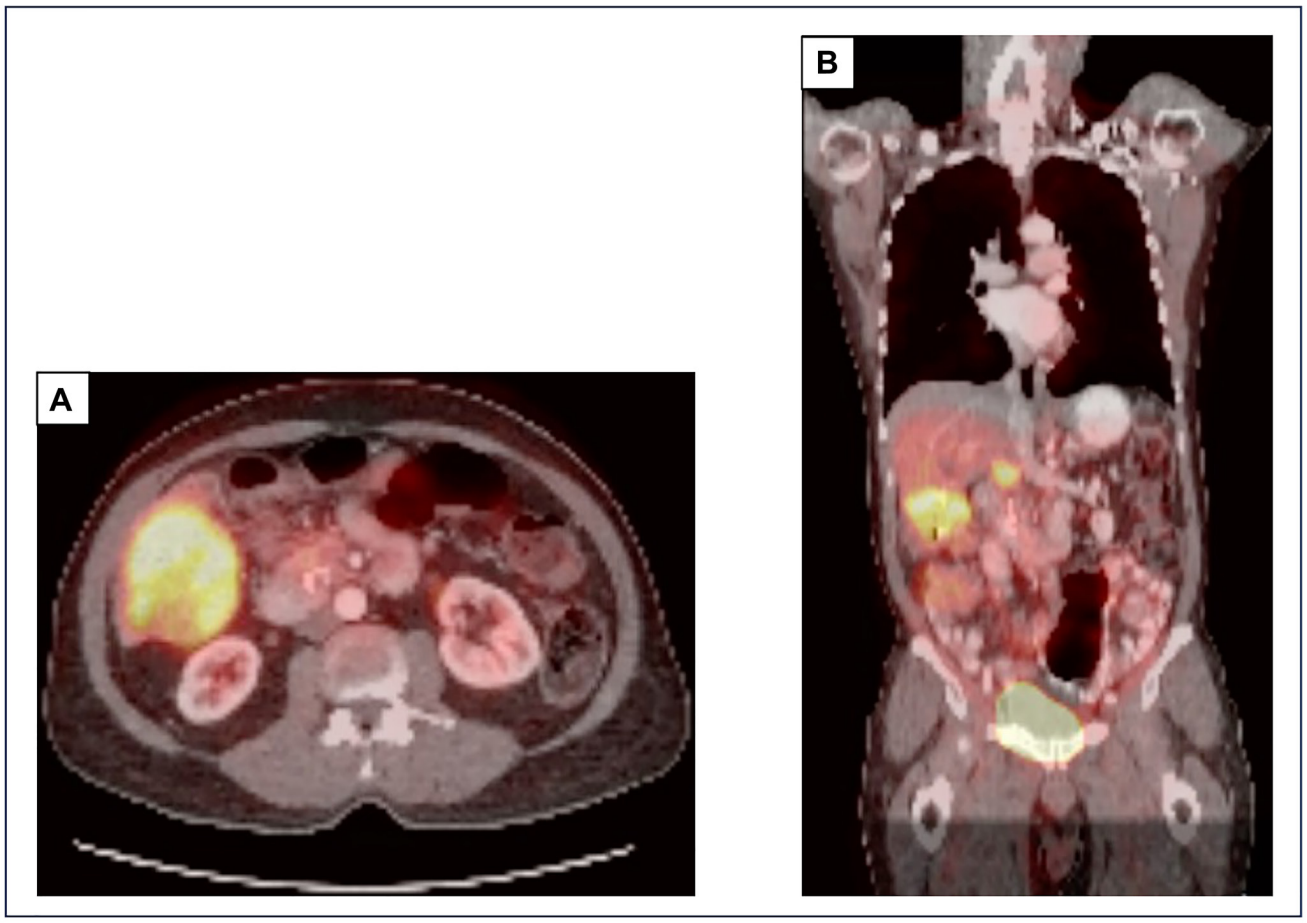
PET-CT scan at our institution prior to staring sotorasib demonstrates an 8.2 cm mass in the right inferior lobe of the liver with a SUV of 10.8 (A, B) and a 2.7 cm pancreatic head mass with a SUV of 5.8 (B). Abbreviations: PET: positron emission tomography; CT: computed tomography.
